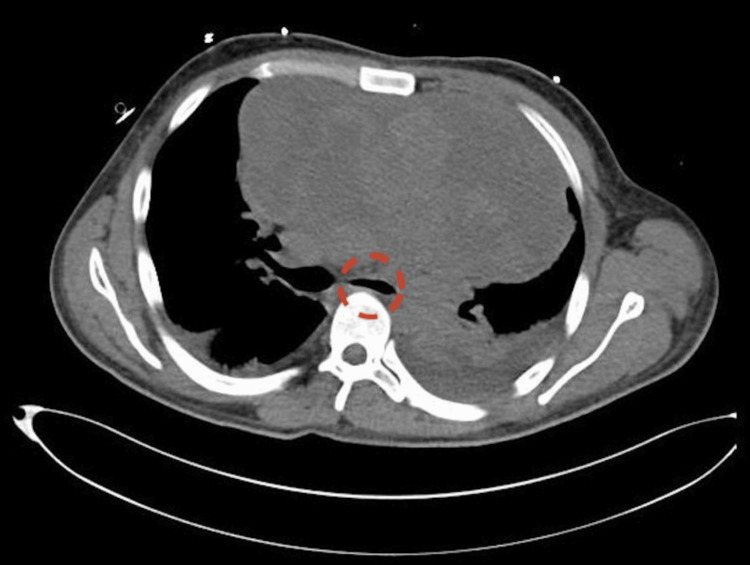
A CT scan of the thorax shows the mediastinal mass with significant compression of the patent left mainstem bronchus (dashed circle).
